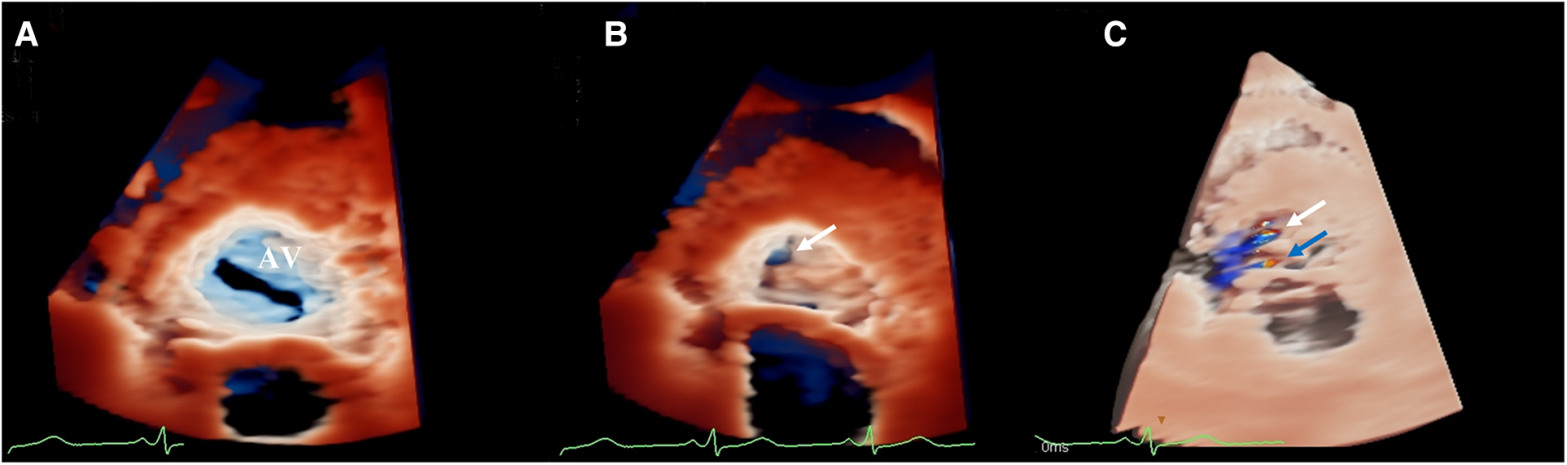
(A) TrueVue vividly revealed a bicuspid aortic valve. (B) TrueVue clearly showed an echolucent area at the ten o’clock position of the left anterior leaflet, suggesting an area of perforation of 0.24 cm^2^ (white arrow). (C) TrueVue merged with three-dimensional color Doppler clearly demonstrated the origin of two different aortic regurgitant jets. One originated from the perforation (white arrow), and the other originated from the prolapsus (blue arrow). AV, aortic valve.
